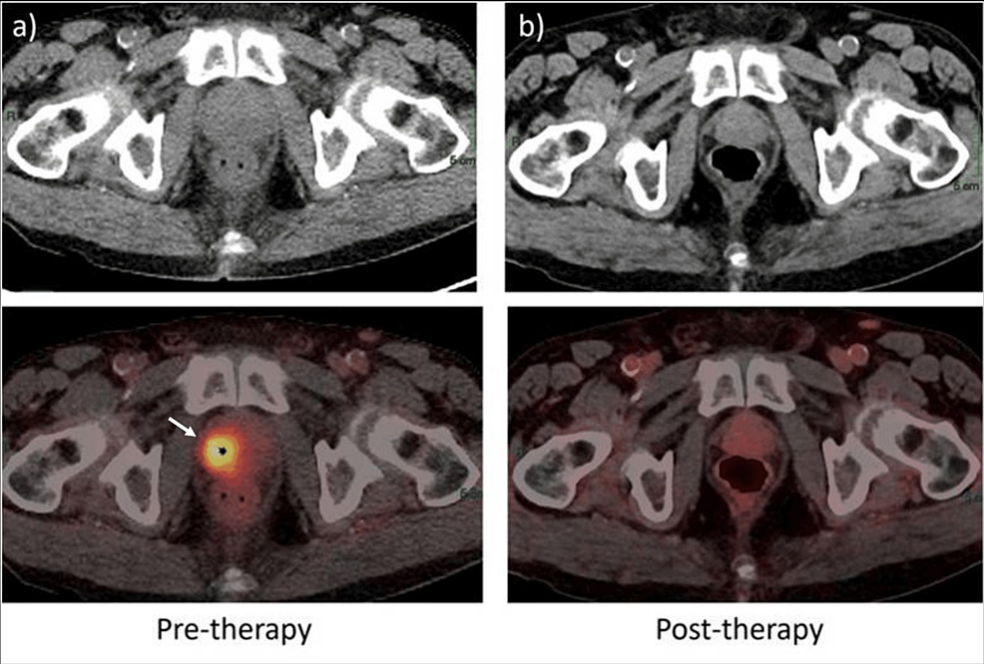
Baseline gallium-68-prostate-specific membrane antigen positron emission tomography/computed tomography (68Ga-PSMA-PET/CT) shows a large tracer-avid lesion in the prostate gland’s right lobe extending up to the seminal vesicles in the midline with standardized uptake value maximum of 12.4 (white arrow and star). Post-therapy 68Ga-PSMA-PET/CT show complete resolution of prior PSMA-avid seen in the prostate gland.
